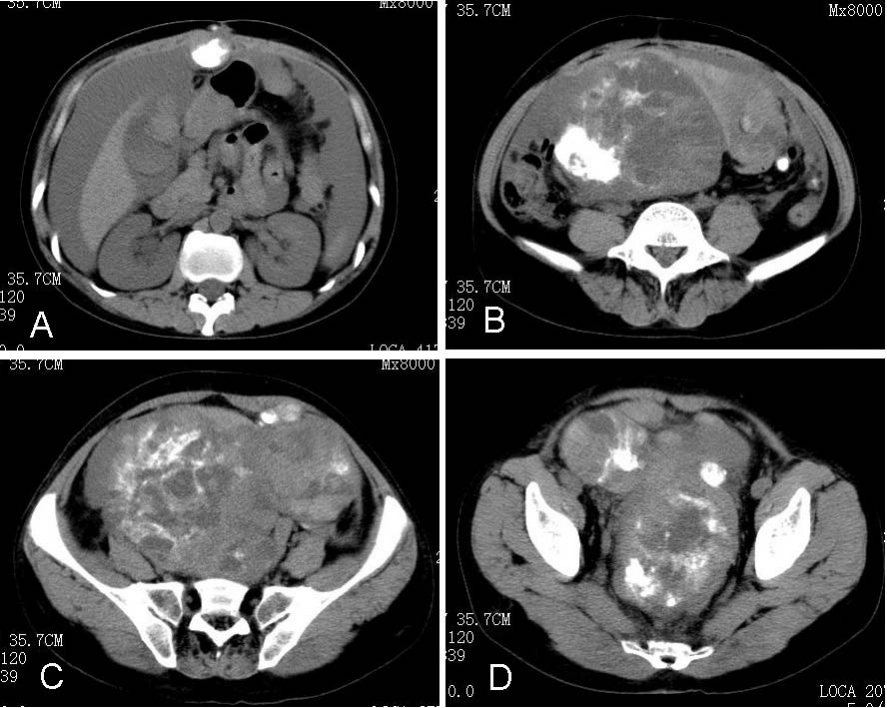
Abdominal CT revealed a large mass with mottled calcification, and effusions in the peritoneal cavity.
